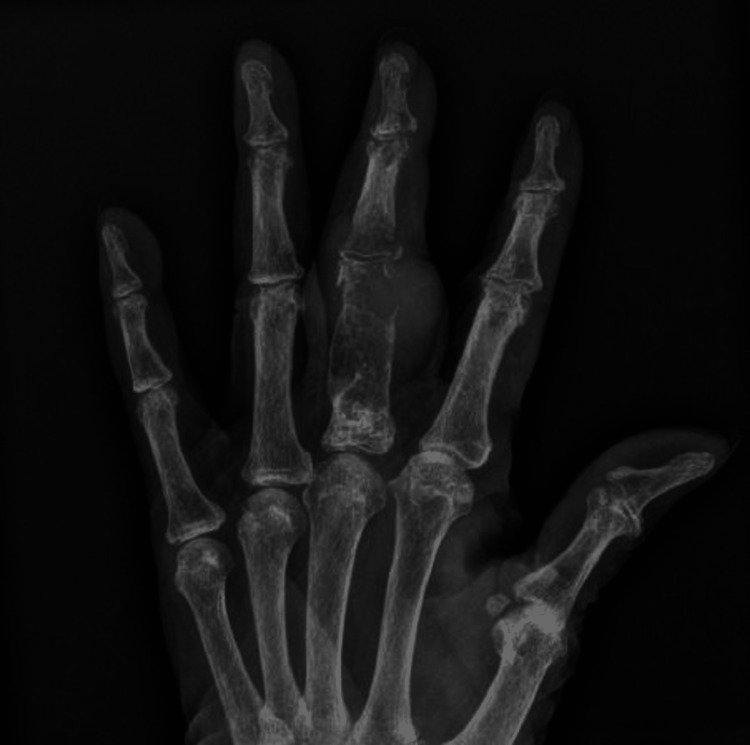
X-ray of the hand showing an expansile lytic lesion with a pathologic fracture of the third proximal phalanx
